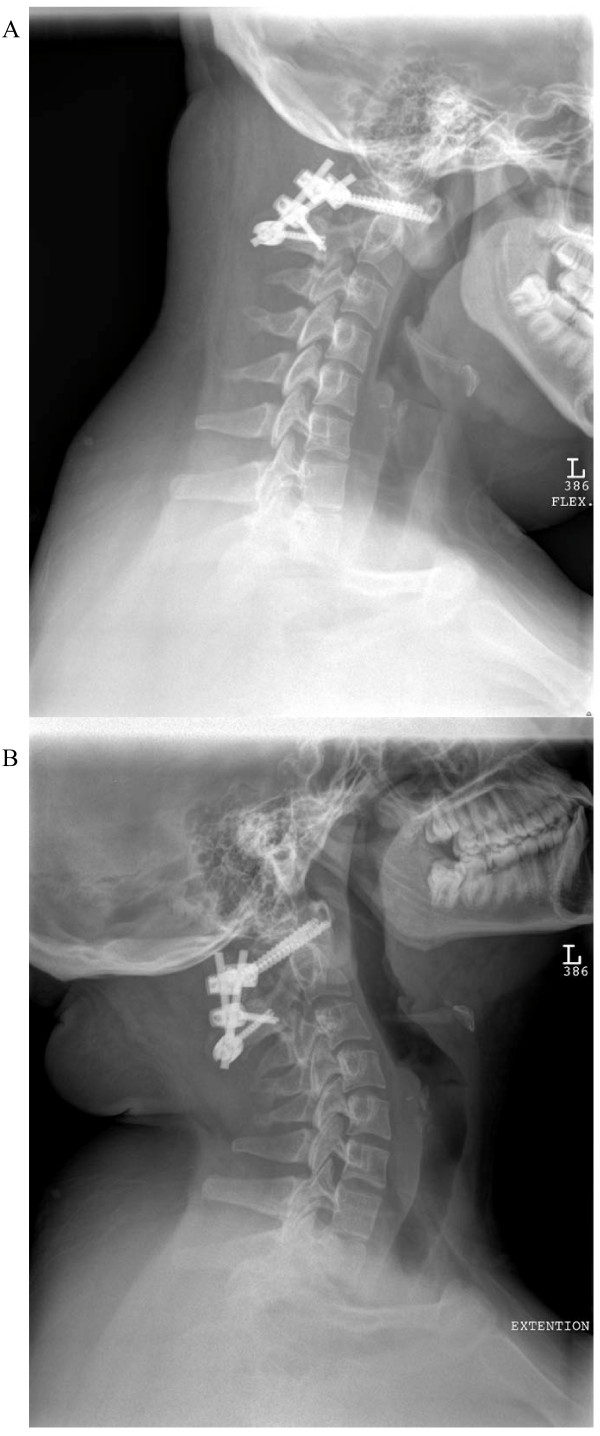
A) Lateral cervical spine X-ray with flexion view at three months after surgery shows no abnormal movement between C1 and C2 vertebrae. B) Lateral cervical spine X-ray with extension view at three months after surgery shows no abnormal movement between C1 and C2 vertebrae.
